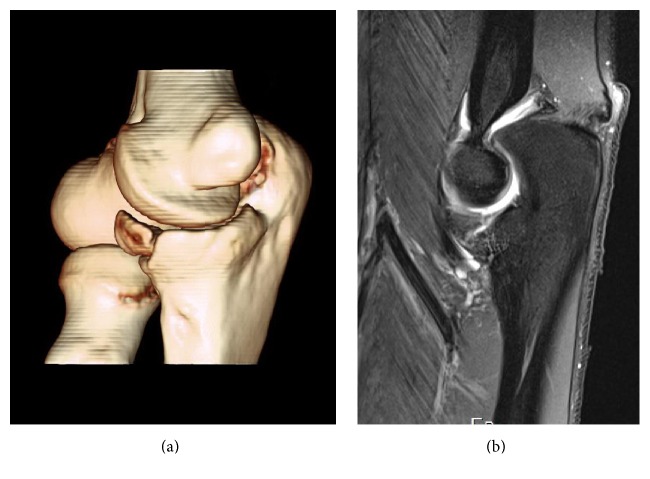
Follow-up imaging studies at 4 and 6 months after diagnosis. (a) Three-dimensional CT-scan reconstruction of coronoid process nonunion: no callus formation and no displacement. (b) Elbow MRI: nonunion and lack of edema or joint effusion.
